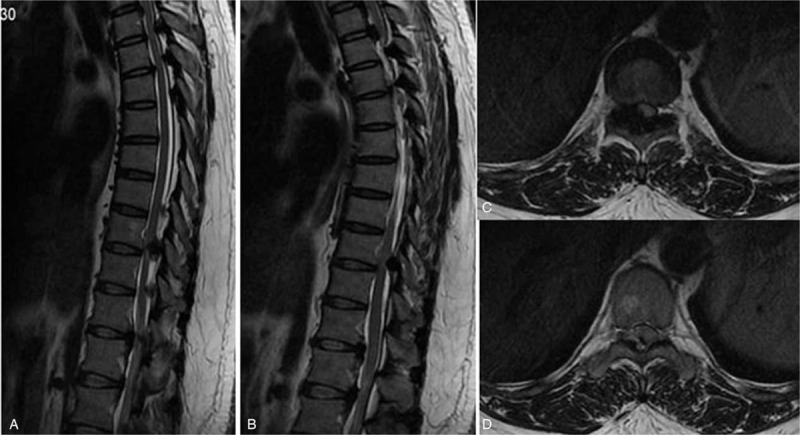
Preoperative magnetic resonance imaging: T9 and 10 ossification of the ligamentum flavum compression of the spinal cord.
